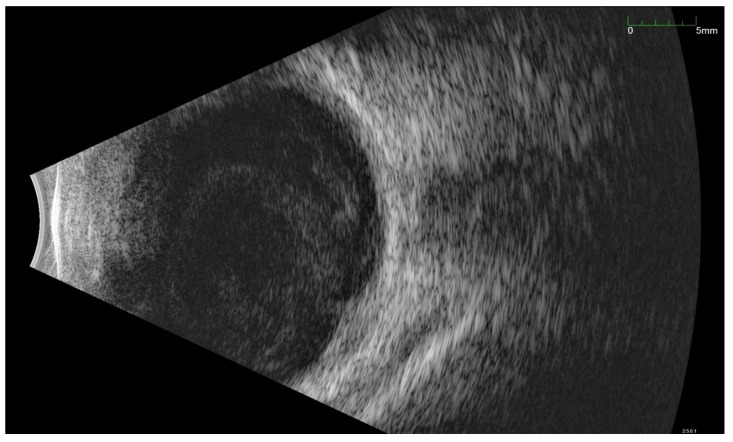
Ultrasonography B scan with bacterial EE. As in Figure 6, hyperechoic densities fill the entire vitreous chamber. However, the lower intensity of the changes is noticeable.
